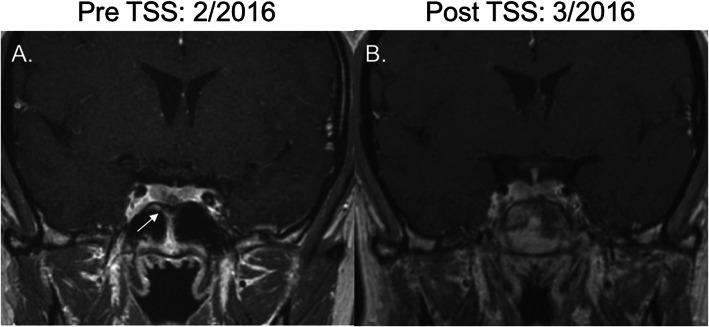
Coronal T1-weighted MRI scans. a. February 2016: Inferior pituitary 5 mm lesion indicative of recurrence of Nelson’s syndrome. Imaging completed prior to third transsphenoidal surgery. b. March 2016: Resolution of pituitary lesion after third transsphenoidal surgery
